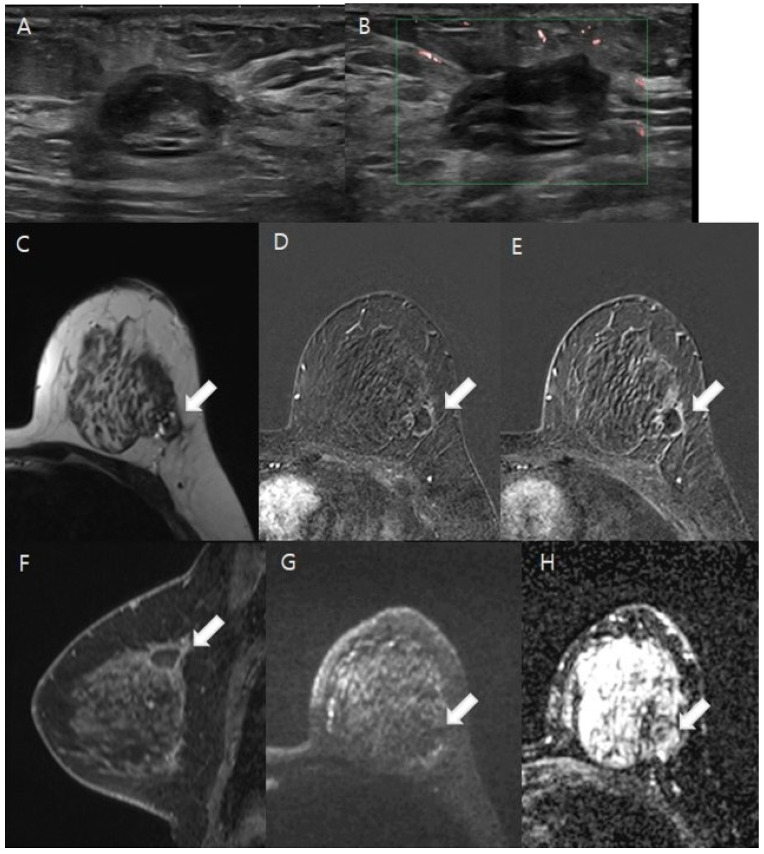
A 41-year-old woman diagnosed with ductal carcinoma in situ (DCIS) after an excisional biopsy. (A,B). The ultrasound reveals an oval hypoechoic lesion with internal hyperechogenicity located in the dependent area of the left upper outer quadrant of the breast. Doppler ultrasound showed no intra- or perilesional vascularity. (C–H). White arrows indicate the lesions in the following images. On T2-weighted imaging, the lesion presented heterogeneous high and low signal intensities, indicative of an organizing hematoma (C). Thin, linear marginal enhancement was apparent in post-contrast axial and sagittal images (D–F). Diffusion-weighted imaging (DWI) at b = 1000 mm^2^/s and the ADC map (b = 0 and 1000 mm^2^/s) demonstrated no diffusion restriction, suggesting the absence of residual malignancy (G,H). The patient then underwent breast-conserving surgery, and the pathology confirmed no residual malignancy in the left upper outer quadrant.
